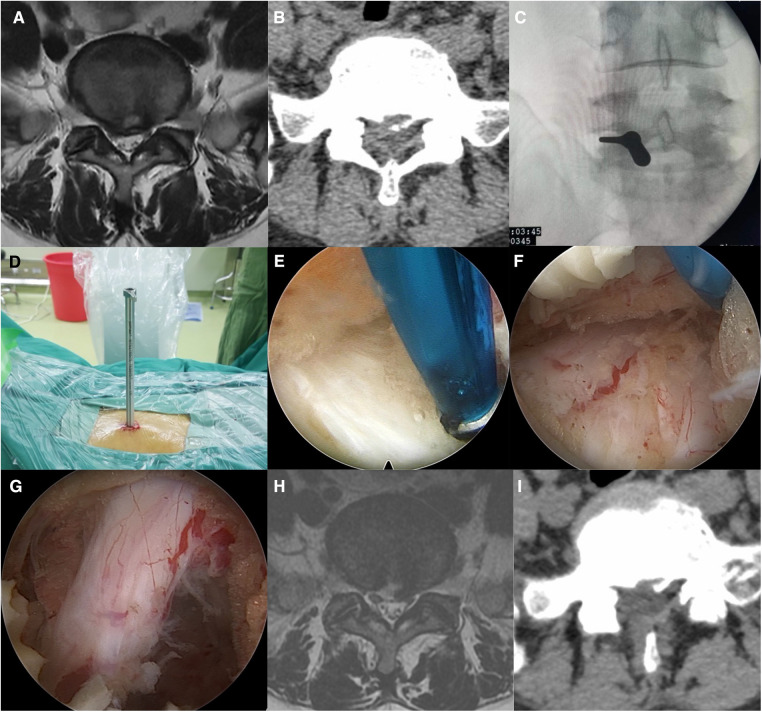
(A,B) Preoperative patient MRI and CT revealing L5/S1 disc herniation. (C,D) Intraoperative positioning and access establishment. (E) Exposure of the interlaminar window. (F) Exposure of the prolapsed disc using a working tube in the axilla of the nerve root. (G) Loosened nerve roots after disc removal. (H,I) Postoperative patient MRI and CT showed that the L5/S1 herniated disc had been removed.
